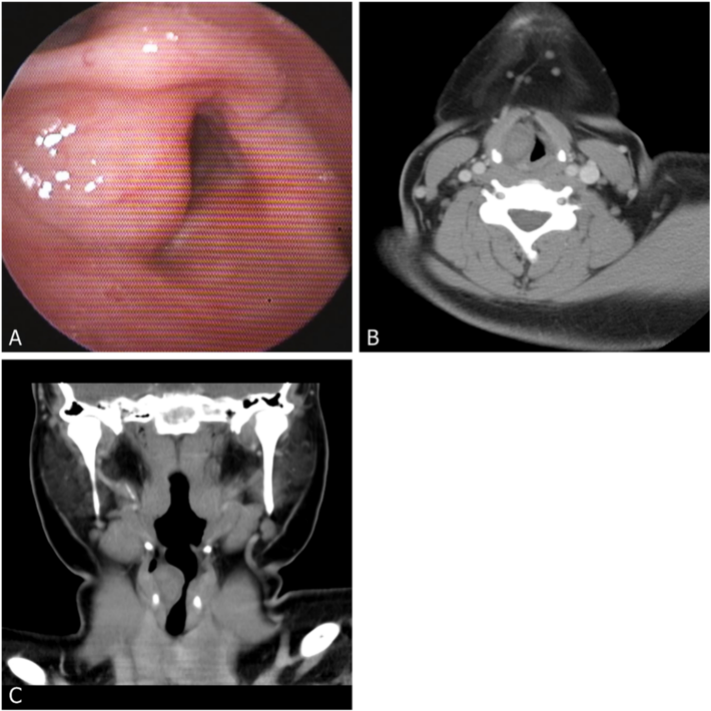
Preoperative findings. (A) Preoperative fiberoptic laryngoscopy revealed a submucosal bulging in the right false and true vocal folds. Preoperative contrast-enhanced computed tomography in (B) axial and (C) coronal view showed a round, slightly heterogenously enhanced mass in the supraglottic area with glottic extension.
